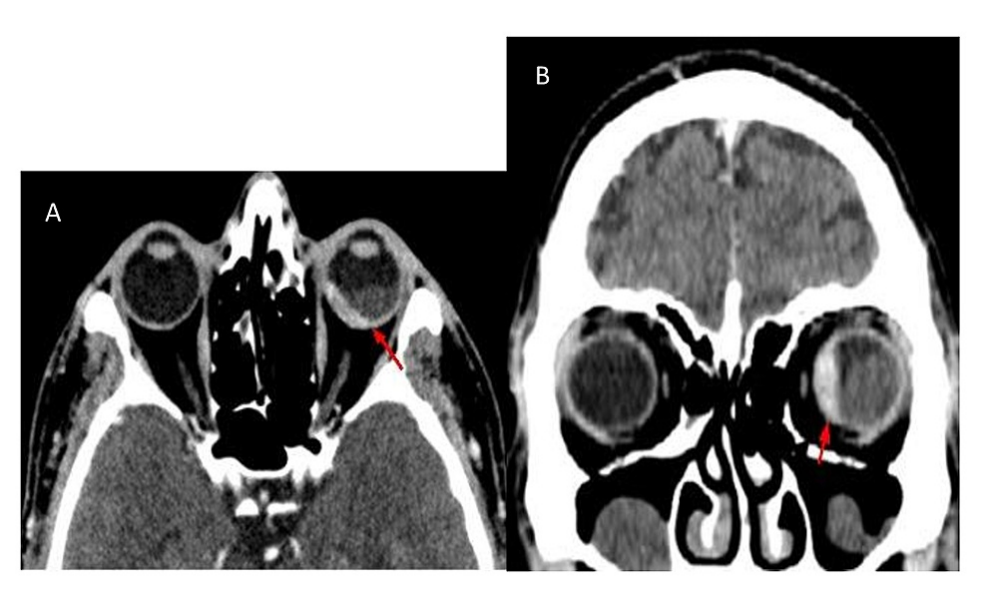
Axial (A) and coronal (B) enhanced CT images showing choroidal thickening of the left eye.
